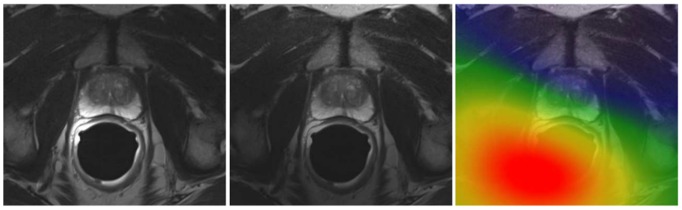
MRI Bias field correction. Left an uncorrected image, in the middle the corrected image and right image with the calculated bias field superimposed.
